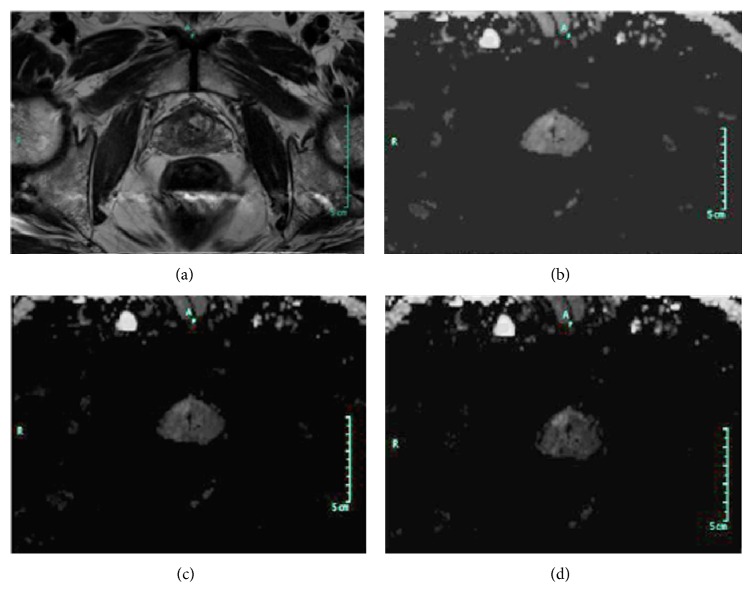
Prostate cancer in a 65-year-old man with a PSA level of 10.5 ng/mL and a Gleason score of 5 + 4. (a) Transverse T2-weighted turbo-spin echo MR image shows the index lesion on midgland, right transitional zone. (b) On cDWI_1500_ image set anteriorly located lesion was barely seen because of inadequate background suppression. (c, d) On cDWI_2000_ and cDWI_3000_ image sets index lesion was more conspicuous. Also a tiny biopsy proven tumoral focus was identified in the right peripheral zone on cDWI_2000_ and cDWI_3000_ image sets.
